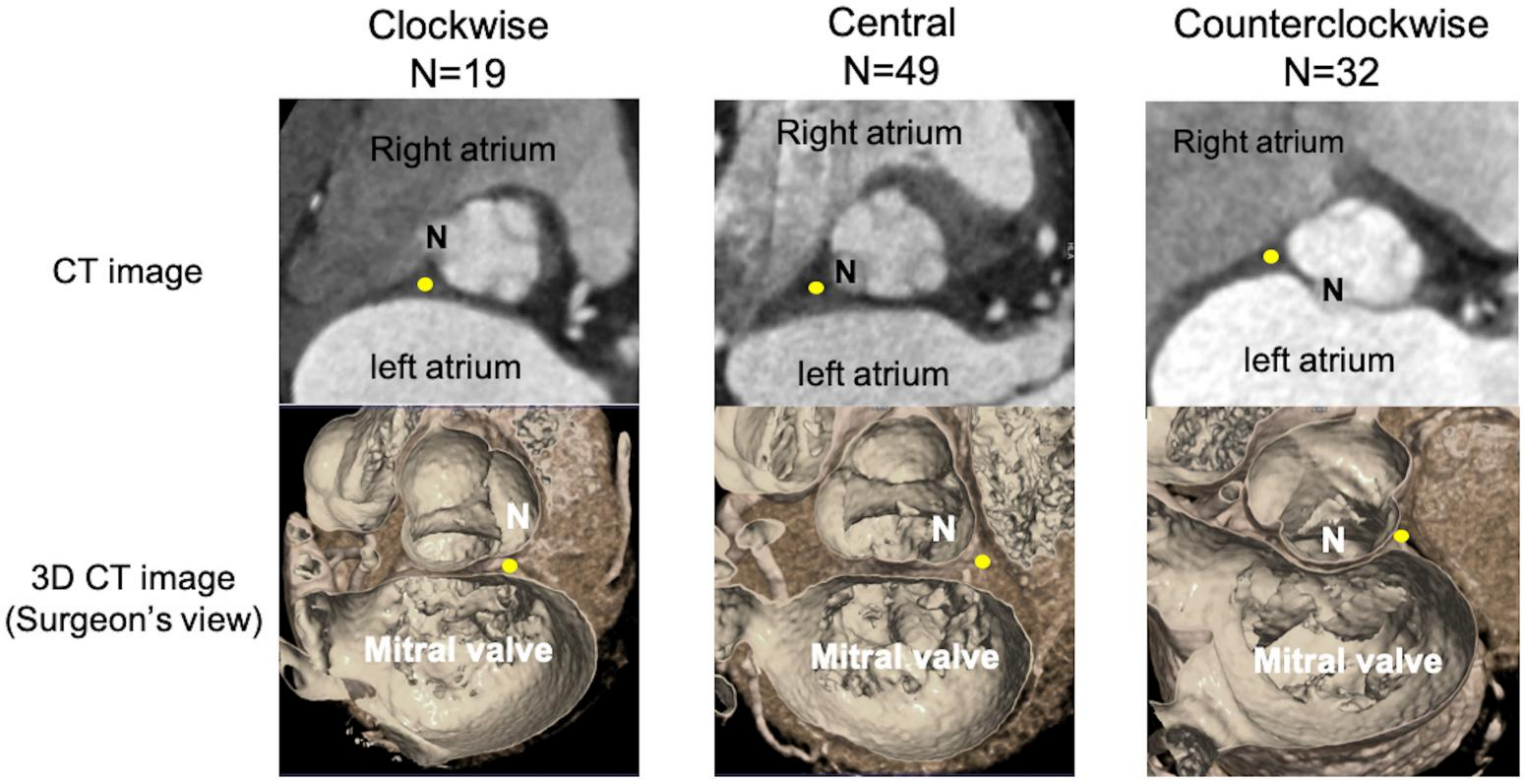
Aortic root rotation classification. Aortic root rotation is classified as clockwise, central or counterclockwise depending on the nadir position of the non-coronary sinus relative to the atrial septum (yellow dots). CT: computed tomography; N: non-coronary sinus; 3D: three- dimensional.
